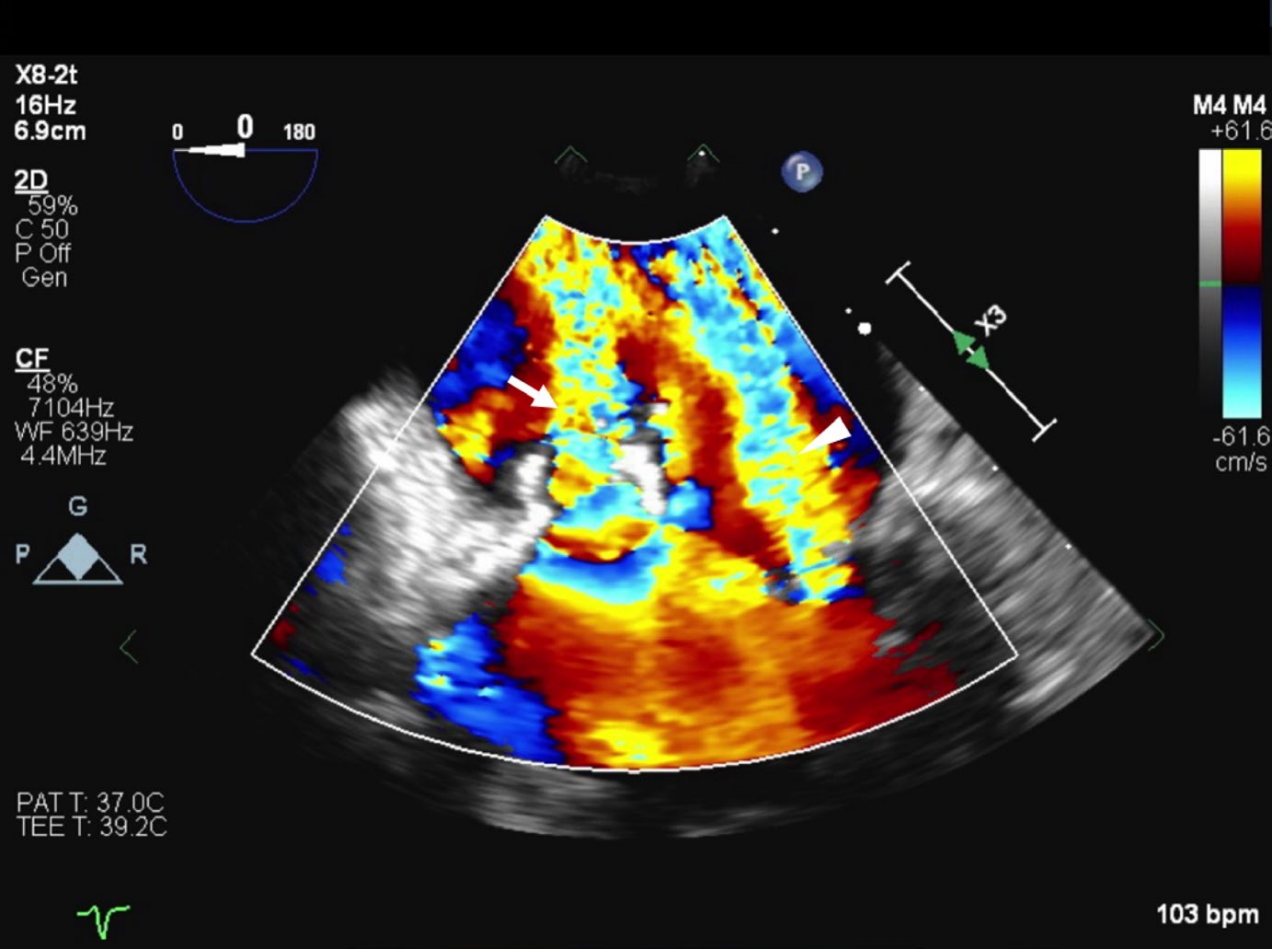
2D transesophageal color flow showing mitral regurgitation through the perforation (arrow) and the mitral orifice (arrowhead)
